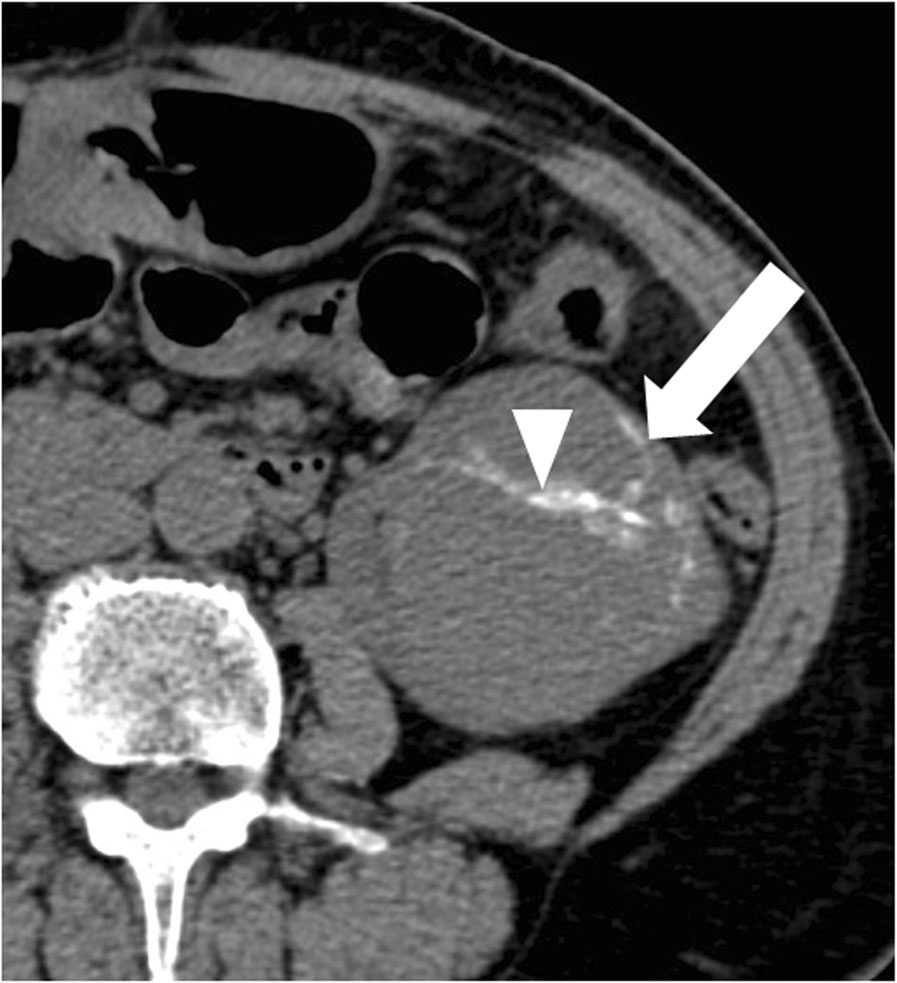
Bosniak category IIF renal cyst. Axial non-enhanced CT image shows a lesion with irregular calcifications within the wall (arrow) and septa (arrowhead)
